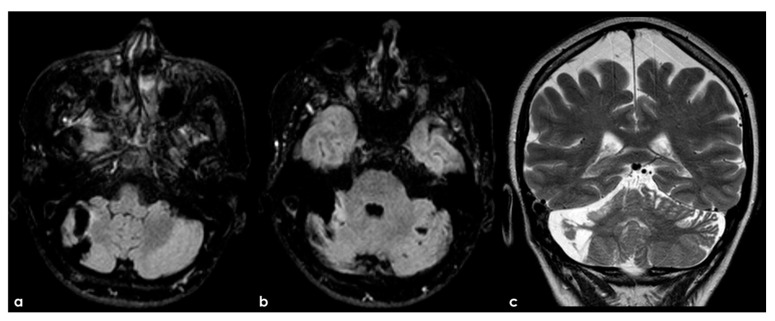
An example of multifocal cerebellar ischemic lesions in a patient with APS (double positivity). Brain MRI (axial FLAIR in (a,b), coronal T2W sequence in (c) shows the poromalacic evolution of multiple ischemic lesions involving both cerebellar hemispheres (right ≥ left). No causes other than APS were identified in this patients.
